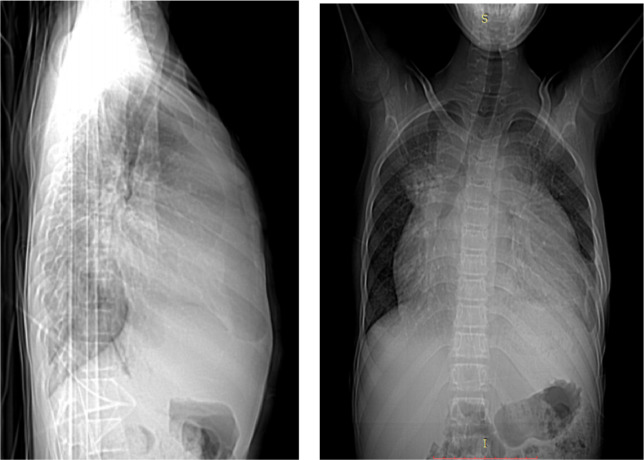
Chest CT-scan showing huge cardiomegaly
